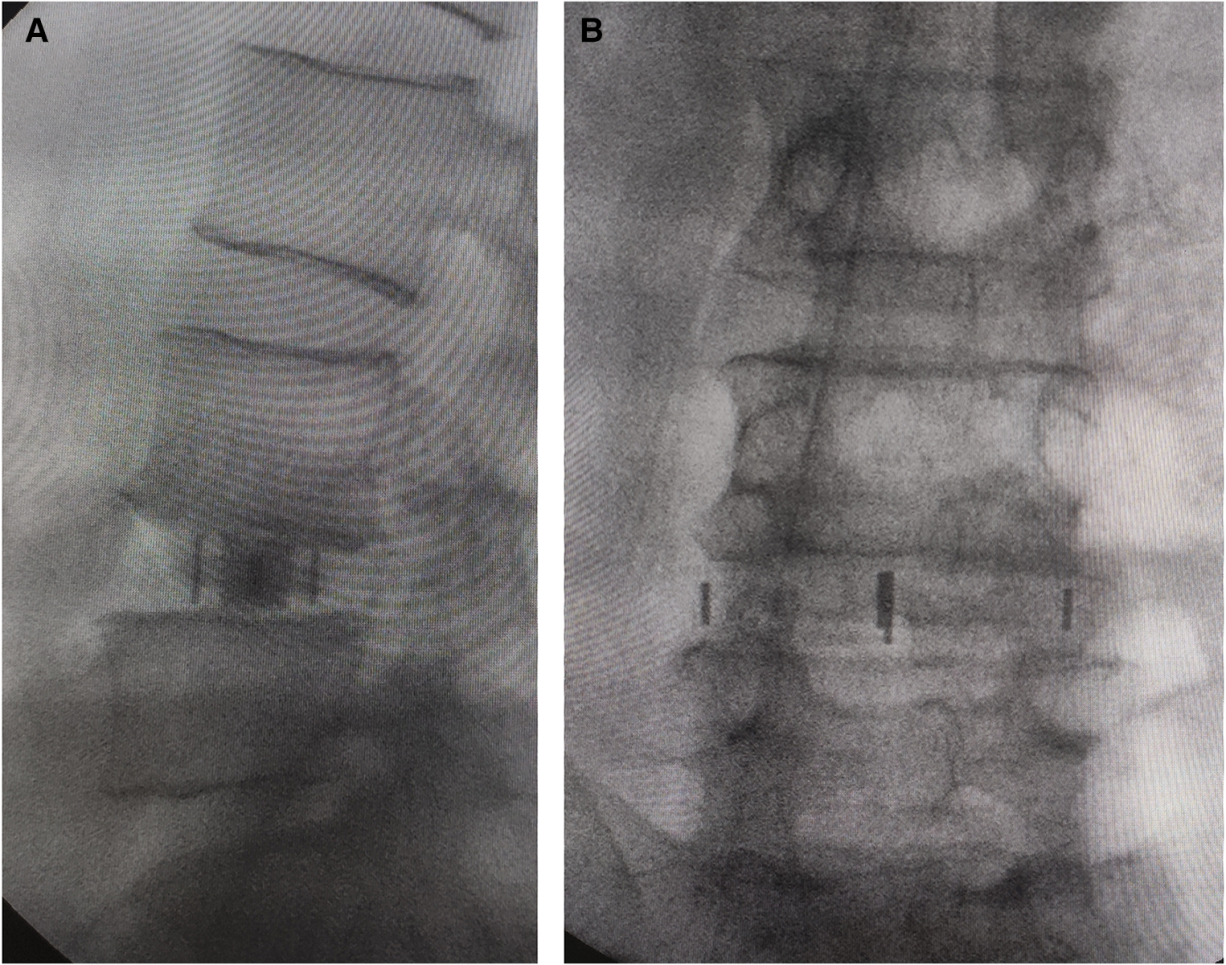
(A) Intraoperative anteroposterior and (B) lateral radiographs to confirm the ideal position of the cage. Anteroposterior and lateral metal markers show the cage was almost perpendicular to the spine. (A) Anteroposterior metal markers show the cage extending the entire width of the disc and vertebral body with support from the lateral aspect of the vertebral body bilaterally.
